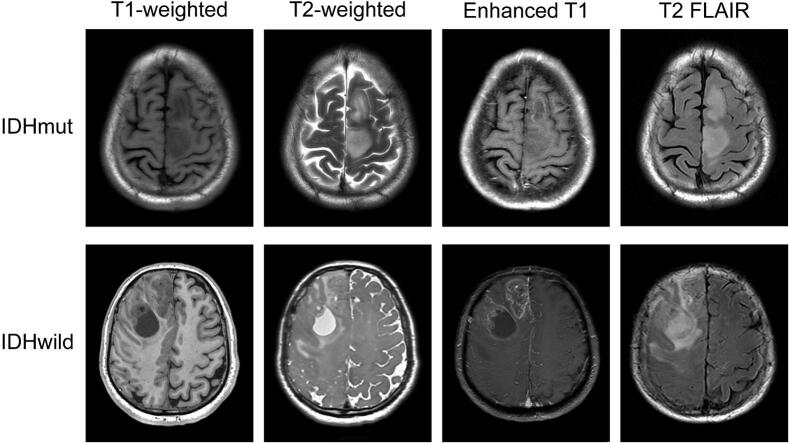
Multiple sequence MRI examples of IDH mutant and IDH wild type multifocal gliomas. IDHmut, IDH mutant type. IDHwild, IDH wild type.
